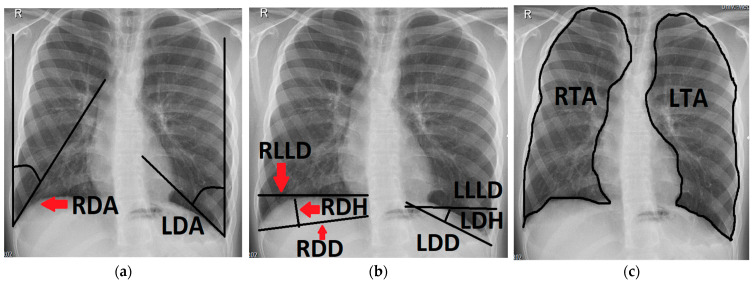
Chest radiograph of a 16-year-old patient after left-sided CDH repair with a cone-shaped patch in the neonatal period; measurements of the following variables are outlined (black line) and labeled on the patient’s right side (red arrows): (a) right and left diaphragmatic angle (RDA, LDA); (b) right and left diaphragmatic diameter (RDD, LDD), right and left diaphragmatic height (RDH, LDH) and right and left lower lung diameter (RLLD, LLLD); (c) right and left thoracic area (RTA, LTA).
